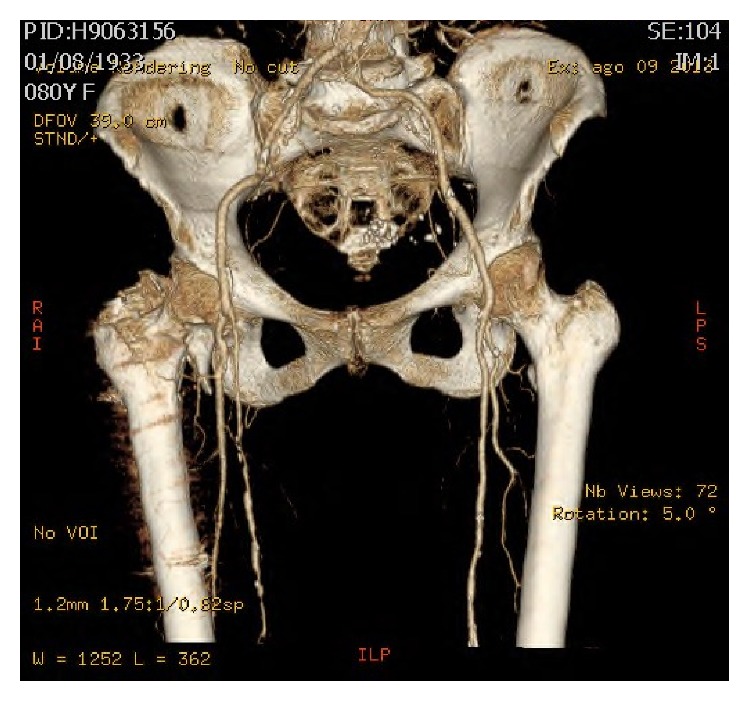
The angio-CT scan demonstrates proximal migration of the lesser trochanter in the femoral triangle of Scarpa without neurovascular involvement.
